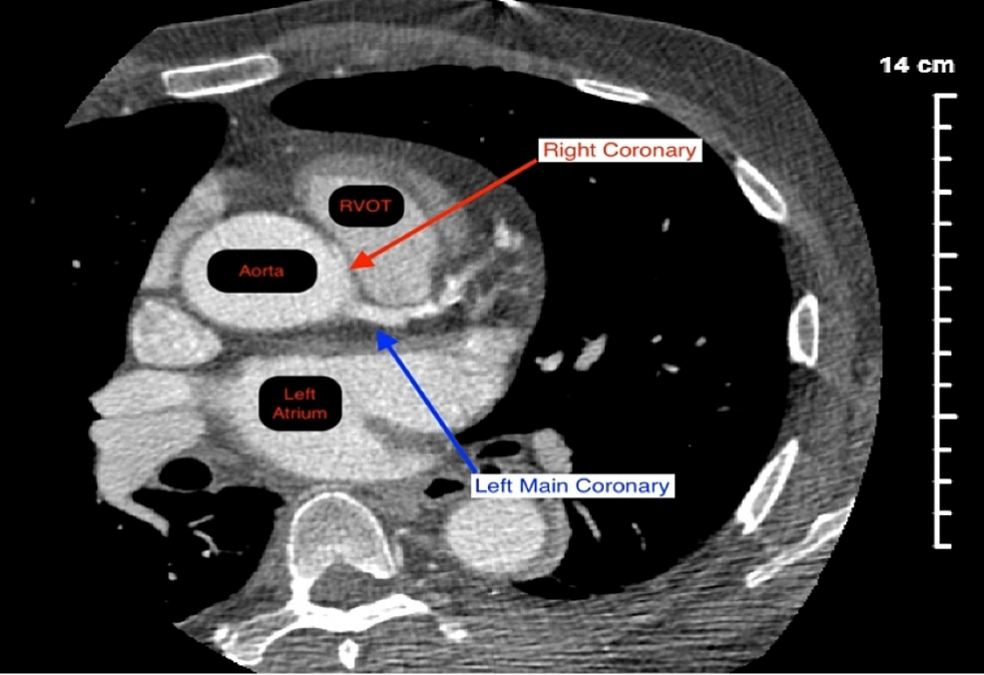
CCTA revealing an anomalous RCA with an acute takeoff angle originating from the left coronary sinus (red arrow) traveling in between the aorta and RVOT. CCTA: coronary computed tomography angiogram; RCA: right coronary artery; RVOT: right ventricular outflow tract
